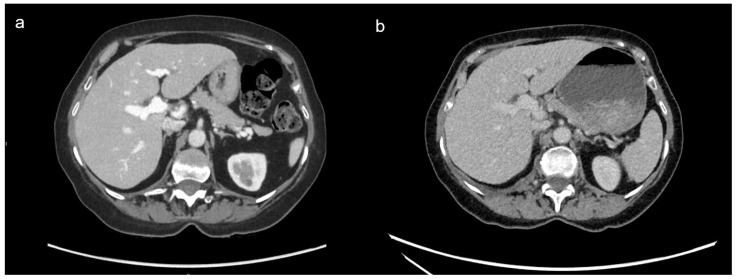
Example of DECT at 60 keV (a) and SECT (b) axial contrast-enhanced CT images of the same patient during the portal venous phase, presented with identical window width (350 HU) and window level (50 HU). VMI_60 keV_ image (left) was rated 5 for both image quality and contrast, whereas the SECT image (right) was rated 3 for both characteristics.
